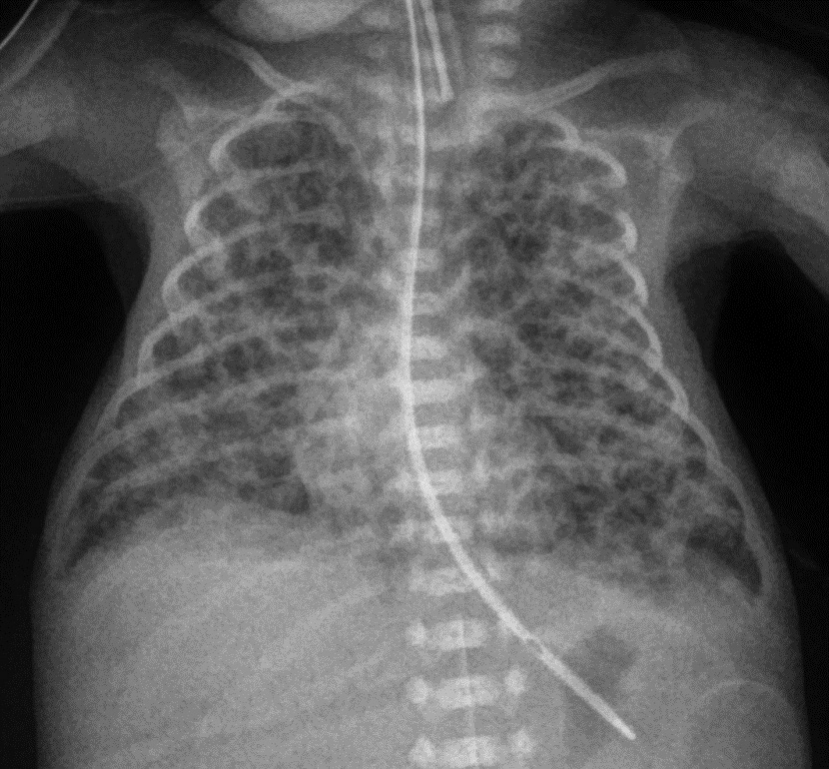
AP chest radiograph 8 days later shows bubbly lucencies in the interstitium throughout both lungs with hazy background opacities. These findings are typical of diffuse bilateral PIE (pulmonary interstitial emphysema) in the setting of RDS.
